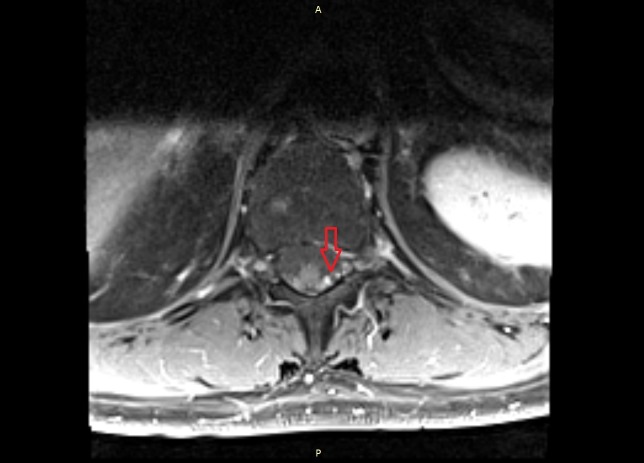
MRI scan. The image shows a probable meningeal carcinomatosis near the thoracolumbar transition. Dorsolateral of the myelon, a contrast-enhancing structure is visible. MRI: magnetic resonance imaging
